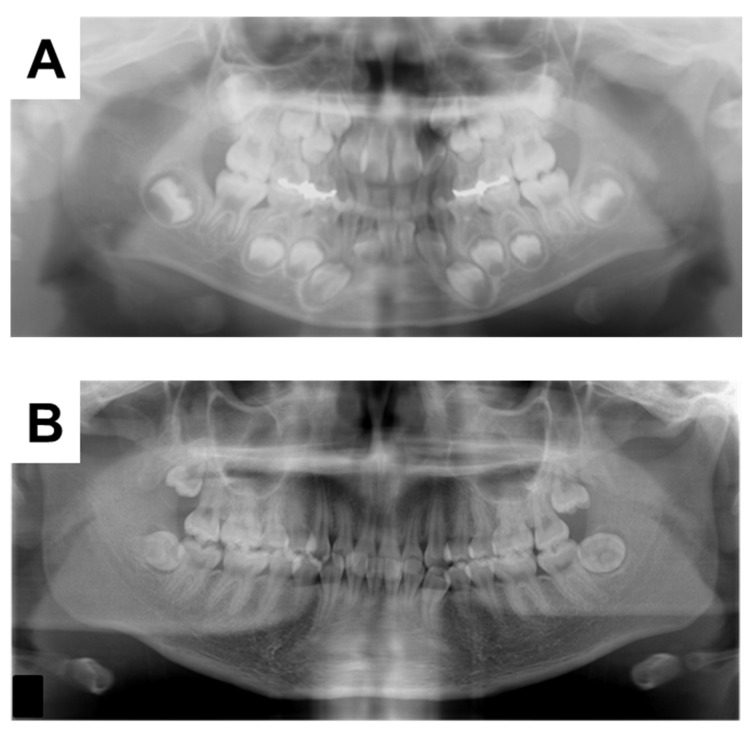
Orthopantomographs taken during the follow-up period at (A) 6Y6M and (B) 17Y5M.
